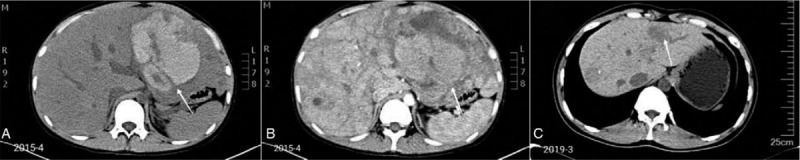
A. A heterogeneous density lesion measuring 13.0 × 8.0 × 13.5 cm was observed in the left hepatic lobe, with an unclear margin. The lesion had uneven density in areas of high central density surrounded by irregular low density. CT values ranged from 15 to 64 HU. B. Dynamic contrast study revealed no enhancement of the lesion. There were another two lesions in the left lateral hepatic lobe and right posterior lobe, measuring 3.4 × 2.2 cm and 2.0 × 1.5 cm, respectively. In contrast, the enhanced arterial phase showed multiple diffuse nodules which were significantly reinforced. The degree of enhancement was in decreasing order in the portal venous phase and the delayed phase. There was no intrahepatic bile duct expansion. C. Low-density lesion measuring 2.0 × 3.0 cm was seen in in left hepatic lobe. There was a patchy slightly high density shadow in the lesion. CT values ranged from 35 to 42 HU.
